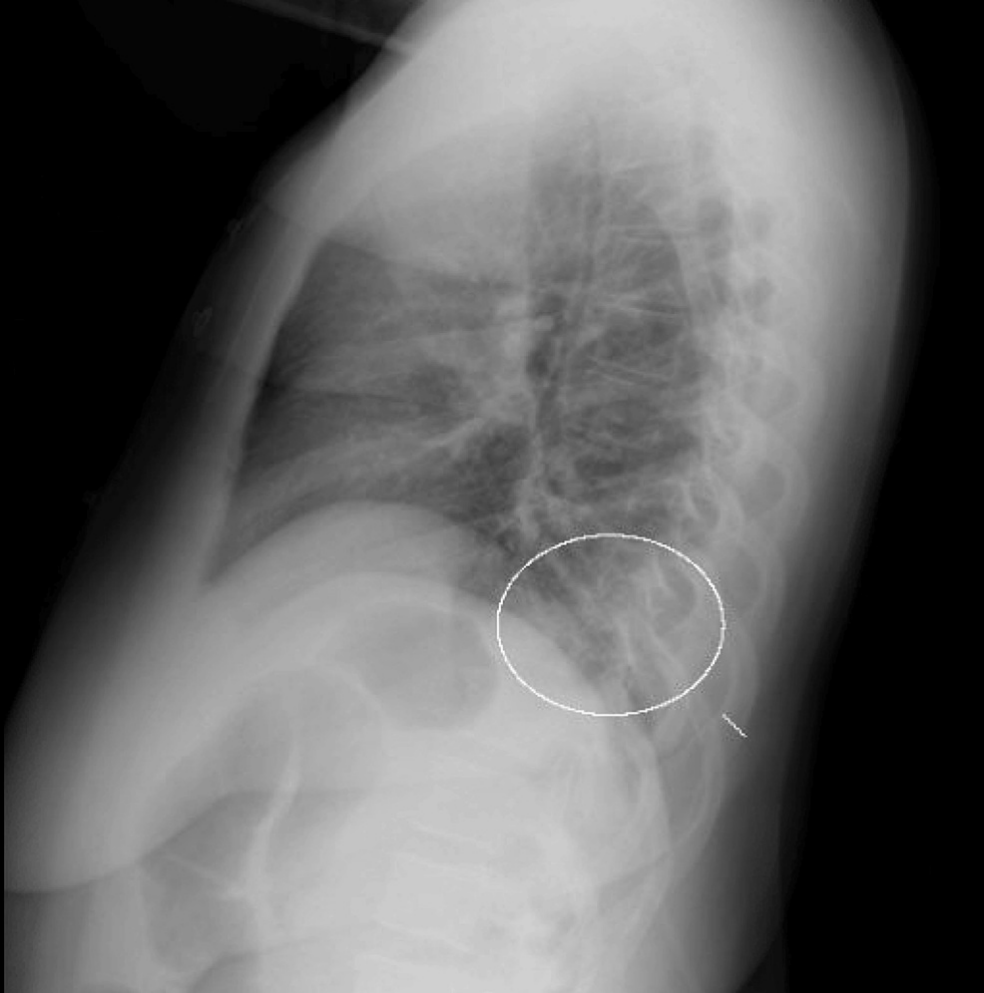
The white circle denotes posterior right lower lobe airspace disease overlapping the right hemidiaphragm. The radiographic findings are suspicious for right lower lobe pneumonia.
